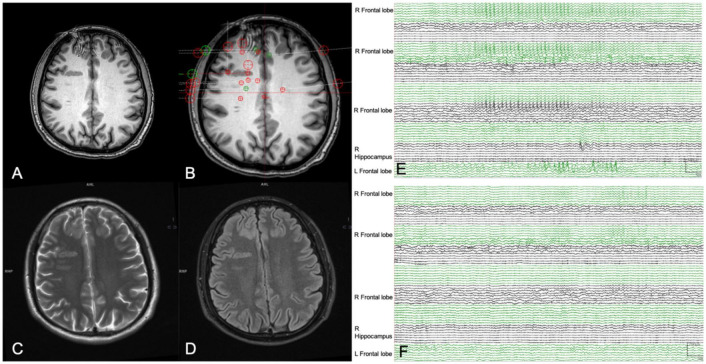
A 26-year-old man (patient #1) with refractory epilepsy for 6 years. Magnetic resonance (MR) imaging axial T1 (A), axial T2 (C), and axial Flair (D) indicated grey matter heterotopia in the right frontal lobe. Stereoelectroencephalography (SEEG) electrodes were implanted to sample all the suspicious seizure onset zone (SOZs) (B). Yet a habitual seizure attack failed to be captured by SEEG. Presurgical interictal SEEG recording (E) showed high-frequency spikes in bilateral frontal lobe and right hippocampus. Combined with semiology and MRI images, the bilateral frontal lobe the right hippocampus was hypothesized to be the suspicious SOZ and stereoelectroencephalography-guided radiofrequency thermo coagulation (SEEG-guided RFTC) was performed. Epileptiform discharges disappeared after RFTC during SEEG monitoring (F). And the patient was seizure-free at 9-month follow-up.
